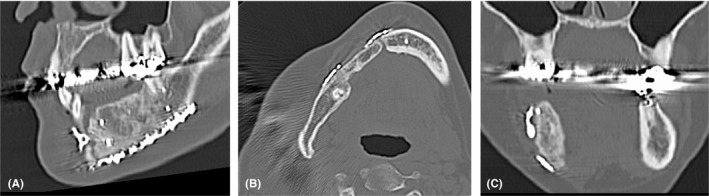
A, Sectional computed tomographic image obtained 1 y and 6 mo after surgery. It can be confirmed engraftment of bone graft. B, The grafted jaw bone is 30 mm high and 10 mm wide. It can satisfactorily accommodate a dental implant of root length. C, The osteosclerosis, similar to the cortical bone, can be identified around the graft bone
